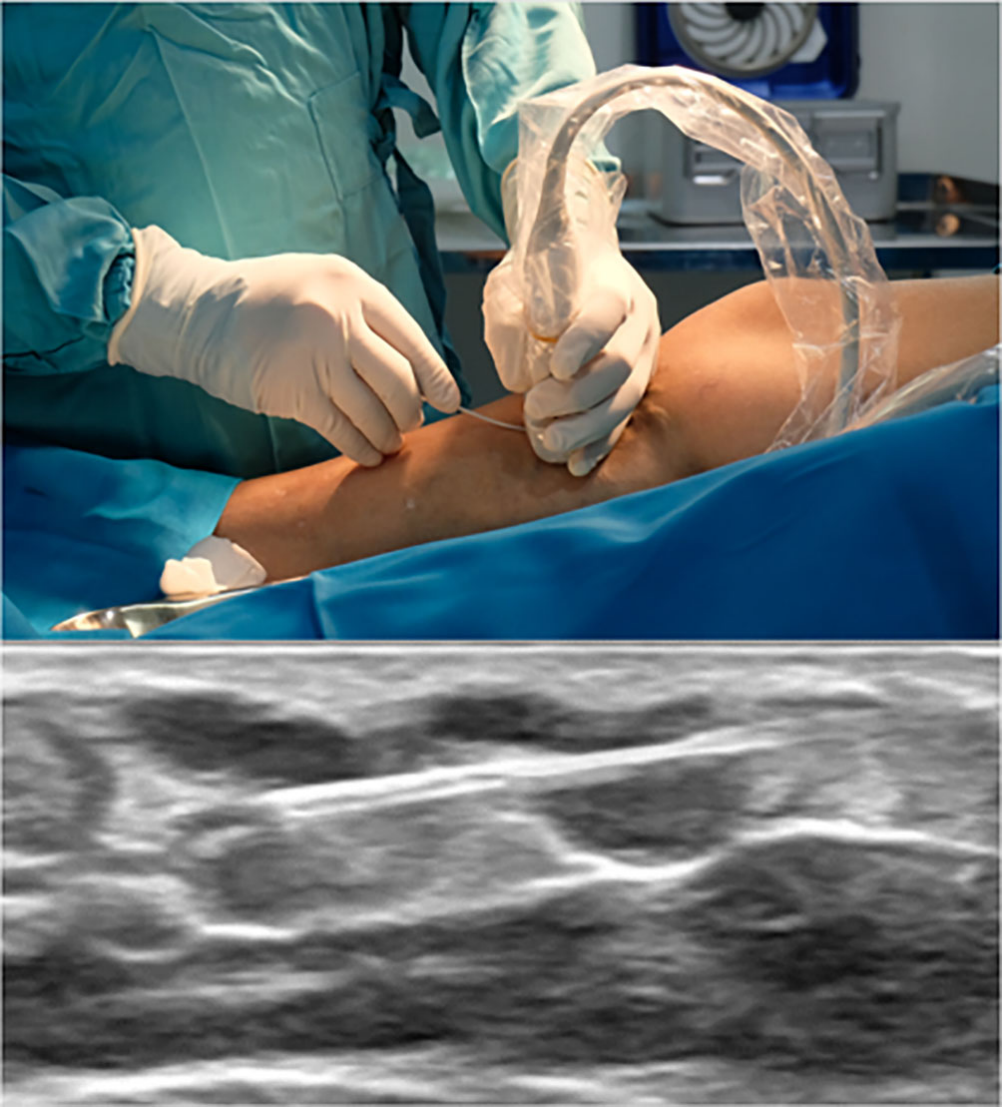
IV Cath Insertion Guided with Ultrasound.
